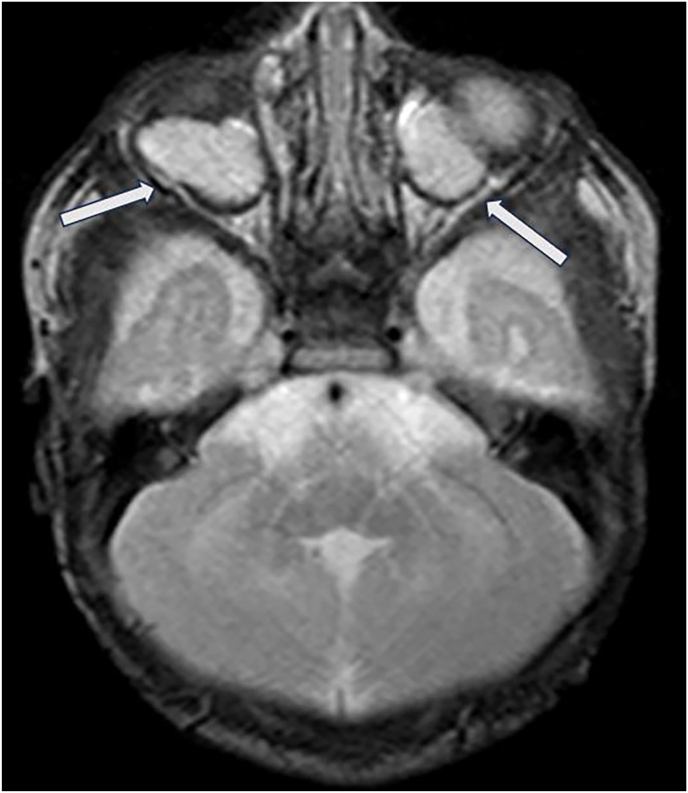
Axial T2 weighted MR image shows retrobulbar cysts (white arrows) with deformity of the globes bilaterally and right microphthalmia.
